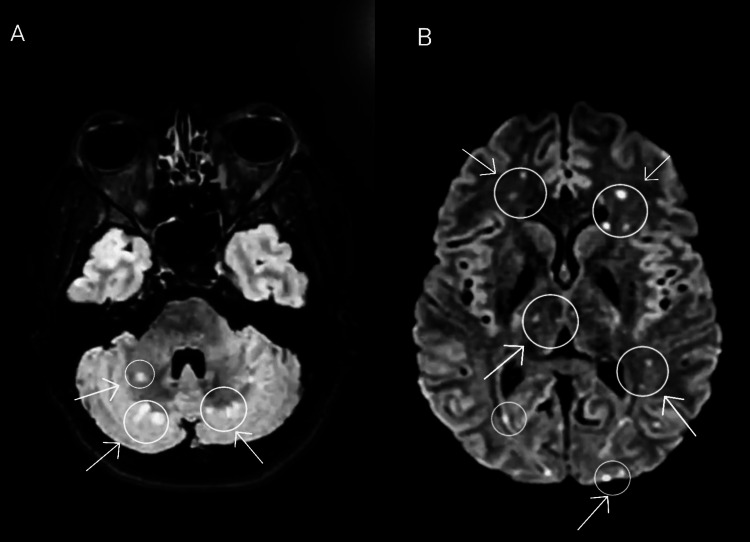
Multiple infarcts were observed in supra and infratentorial areas on brain MRI.
